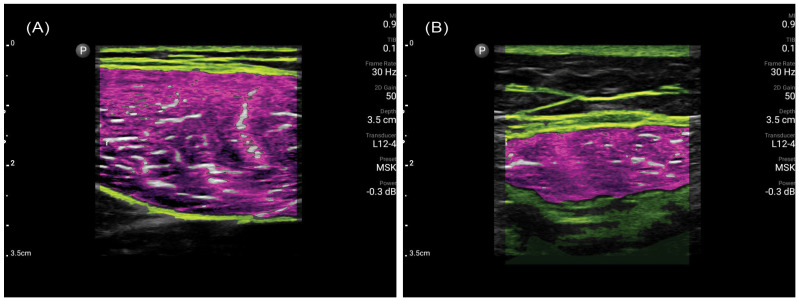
Typical ultrasound images obtained of the rectus femoris muscle after analysis by MuscleSound^®^. (A) is a scan from a 27-year-old volunteer, and (B) is from an 85year-old patient. The pink overlay represents the muscle, yellow represents the muscle boundary and white highlights are non-contractile fibers (in color for print).
